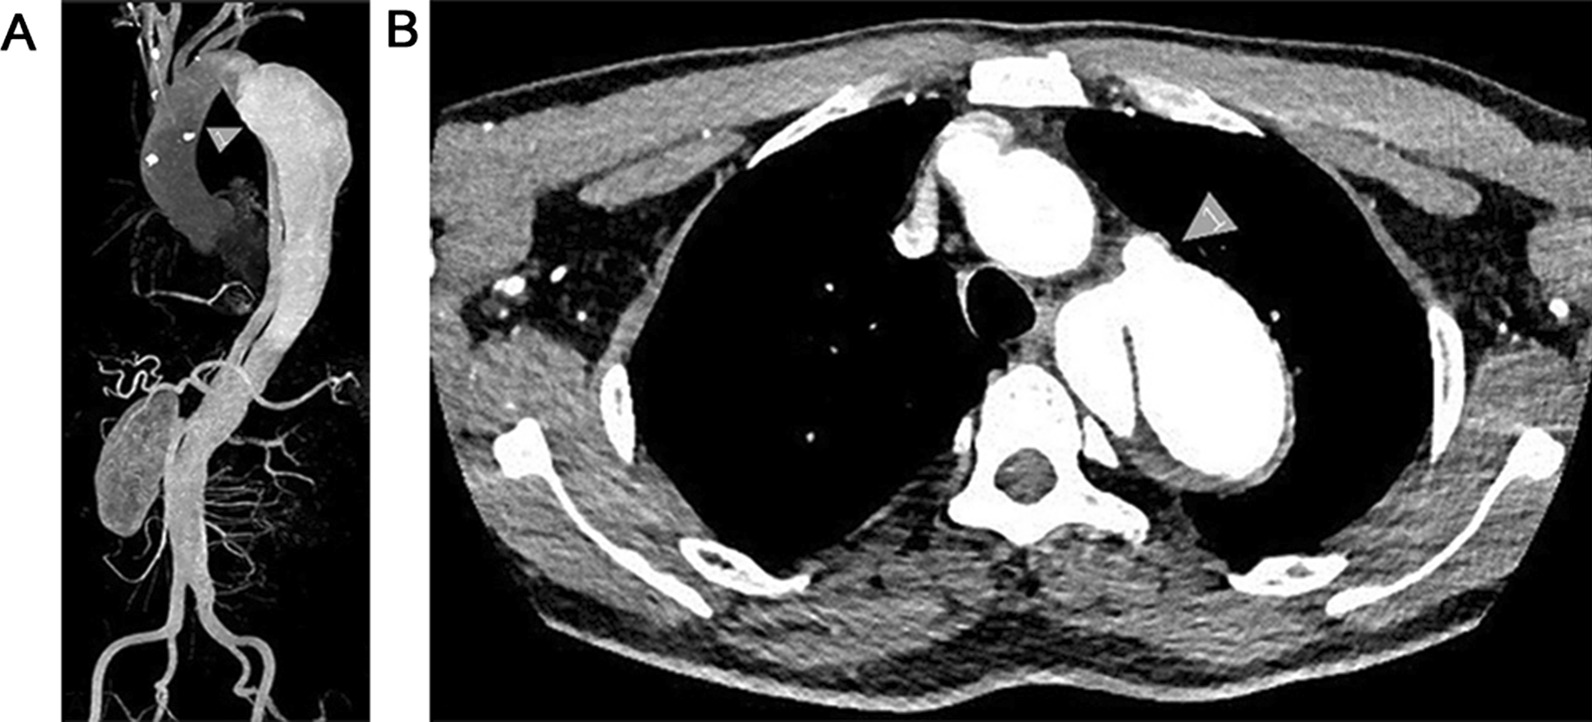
Preoperative CTA was used to determine the anatomical morphology of the patient's lesion. a It can be seen that the false lumen was huge and involved the whole descending thoracic aorta, and the true lumen was severely compressed. b Arrow 1 marked the false lumen
